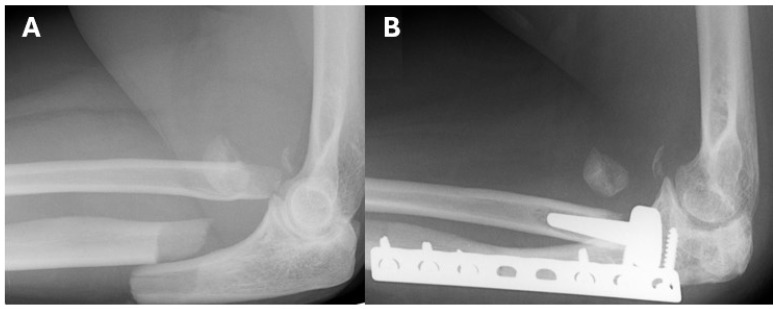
43-year-old female patient with a comminuted fracture of the radial head and a proximal ulna fracture, who underwent fixation of the ulnar fracture with a plate and screws, and implantation of a monobloc radial-head prosthesis. (A) Lateral radiograph of the right elbow before surgery in 2007. (B) Lateral radiograph in 2018 .In both radiographs (A,B), fragments of the radial head that were left during surgery can be seen.
